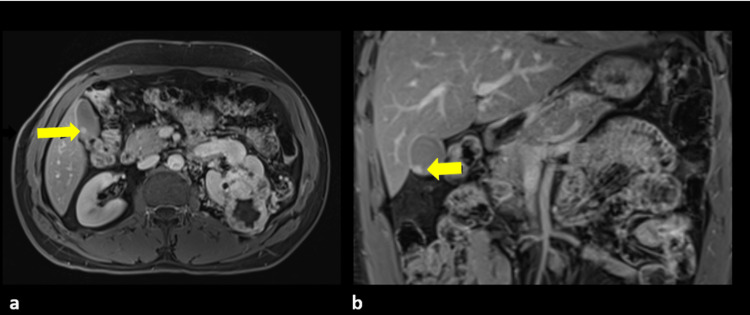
Multiparametric MRI axial (a) and coronal (b) images show an enhancing left renal mass, a tiny enhancing intraluminal gall bladder lesion (yellow arrows) MRI: magnetic resonance imaging
